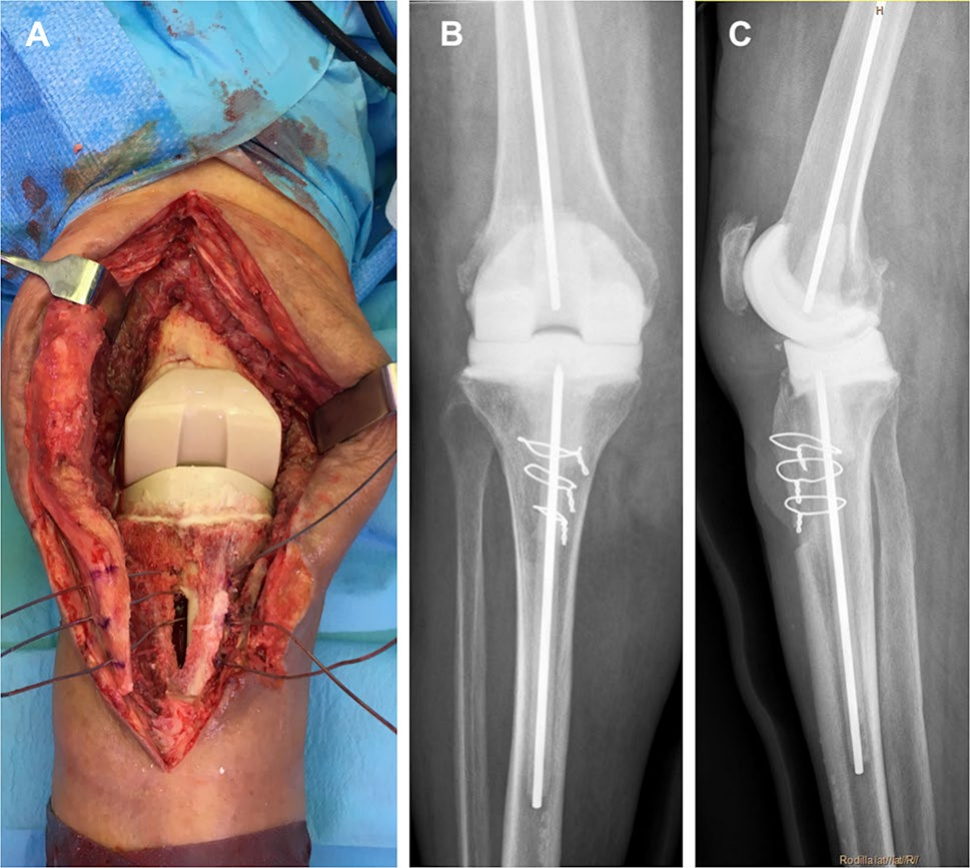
a First stage tibial tubercle osteotomy (TTO) approach. The cerclages pass behind the spacer stem; b anteroposterior and c lateral radiography of a prefabricated mobile spacer
